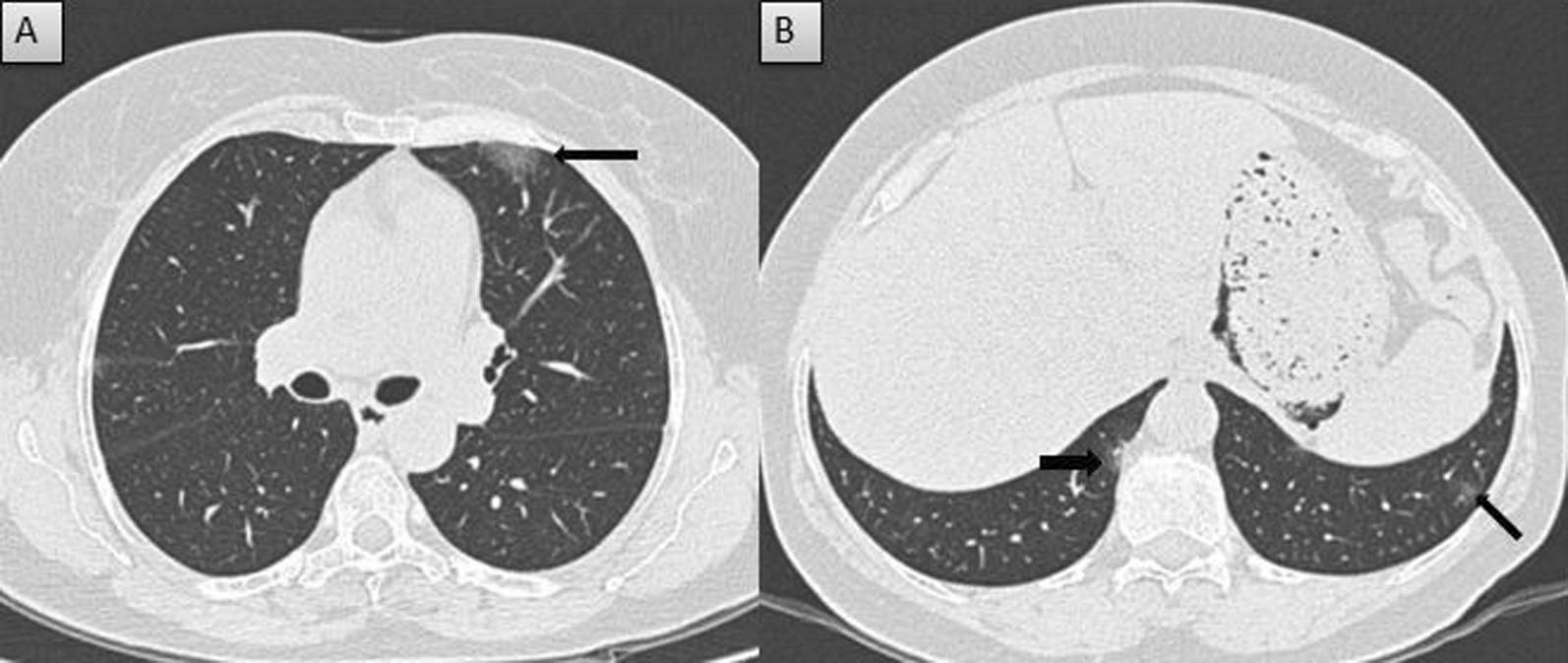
Mild severity in completely vaccinated patient: A and B Axial CT thorax (lung window) of a 59-year-old COVID pneumonia patient who had received two doses of Covid vaccine shows patchy peripheral ground glass opacities with CT severity score of 3/25 (mild severity)
